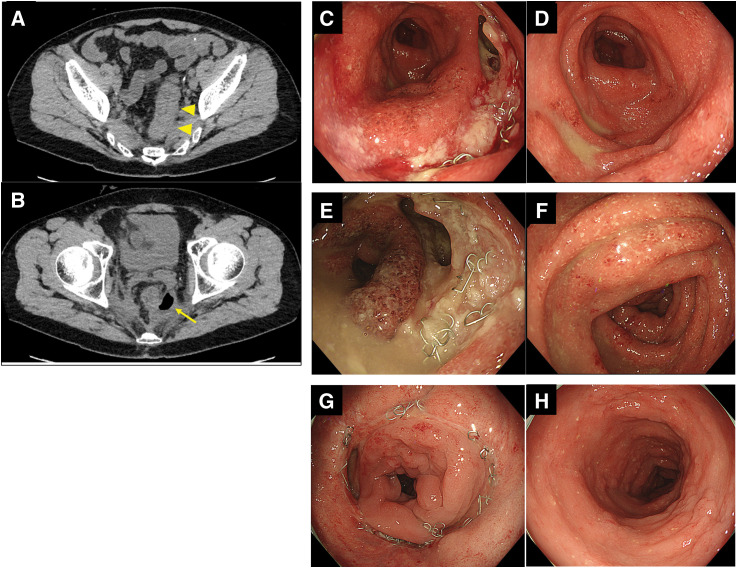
Imaging and endoscopic findings during readmission. (A) CT showing edematous wall thickening of the proximal bowel at the anastomotic site in the descending colon (arrowheads). (B) Fluid collection containing air adjacent to the anastomosis without evidence of free intraperitoneal air (arrow). (C) Colonoscopy on admission demonstrating a deep ulcer at the anastomotic site. (D) Diffuse erythema with purulent erosions in the proximal colon. (E) Repeat colonoscopy on Day 5 post-hospitalization showing progression of the anastomotic ulcer. (F) Worsening inflammatory changes in the proximal colon. (G, H) Follow-up colonoscopy 9 days after initiation of intravenous corticosteroid therapy demonstrating marked healing of the anastomotic ulcer and resolution of inflammatory changes.
